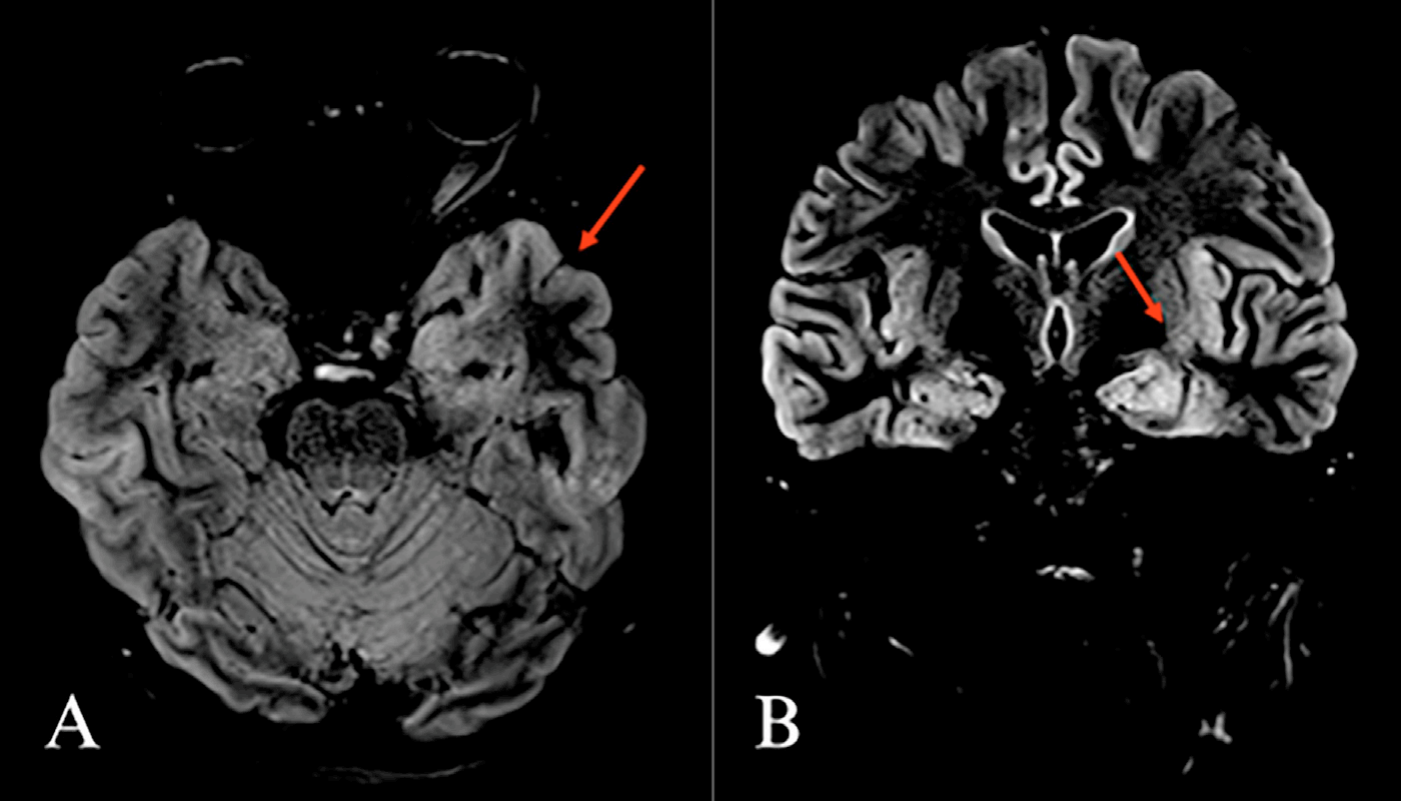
(A) T2-weighted fluid-attenuated inversion recovery (T2-FLAIR) magnetic resonance imaging (MRI) of the head shows subtle increased signal intensity (red arrows) along the inferior aspect of the left temporal lobe on axial and (B) coronal views.
